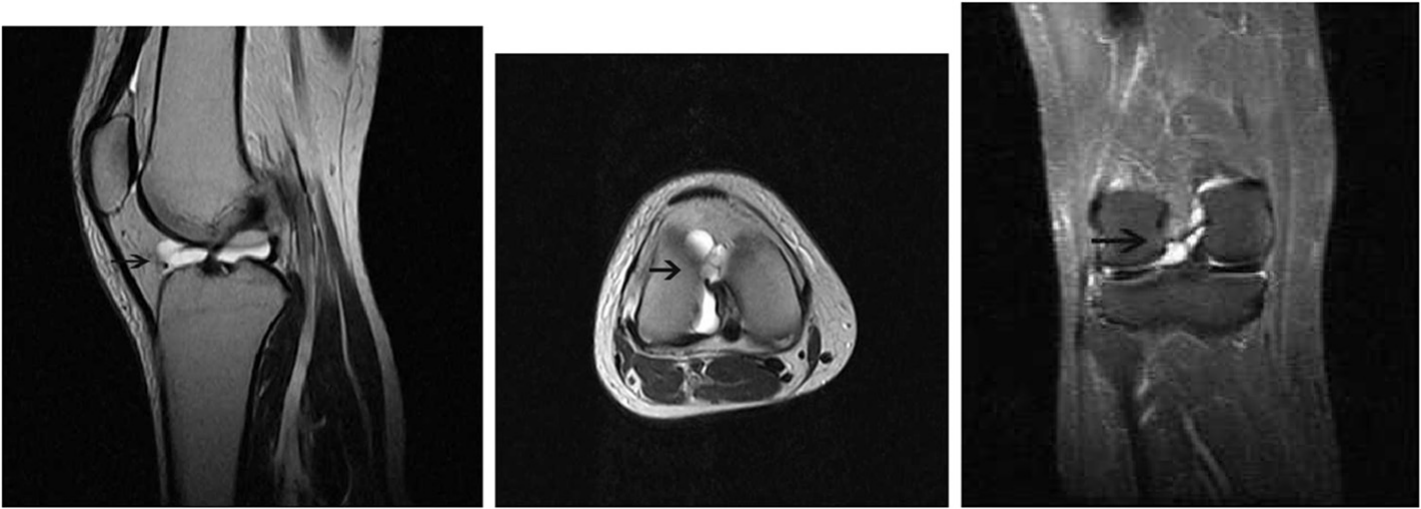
T2-weighted MRI images of the right knee show the multi-lobulated cyst arising from the anterior horn of the medial meniscus to the lateral region of anterior cruciate ligament (black arrow).
